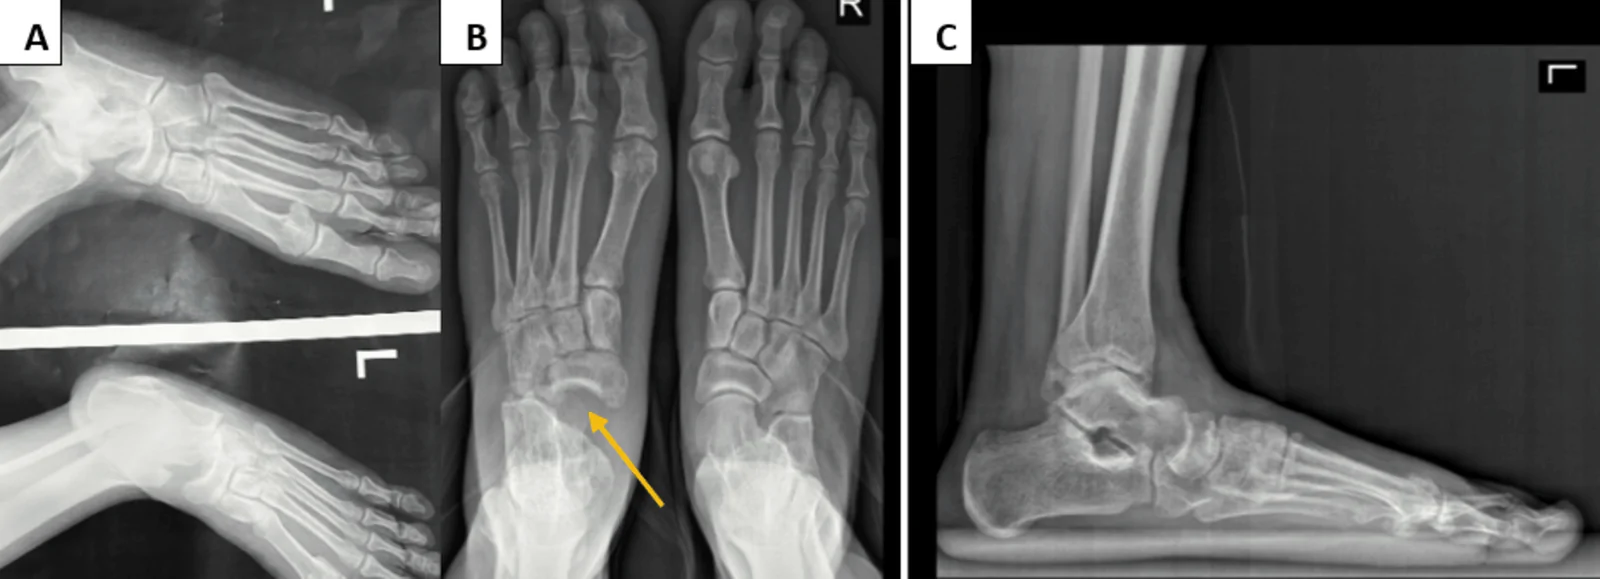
Preoperative radiographs taken 40 days after injury (A) Oblique view, (B) anteroposterior view, and (C) lateral view (arrow showing left foot medial swivel injury)
